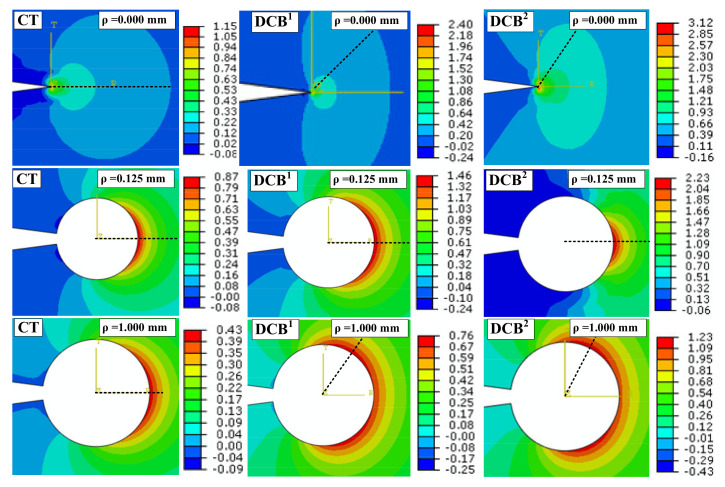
TS contours around VO-notches for CT and DCB models with various radii (γ = 15°).
